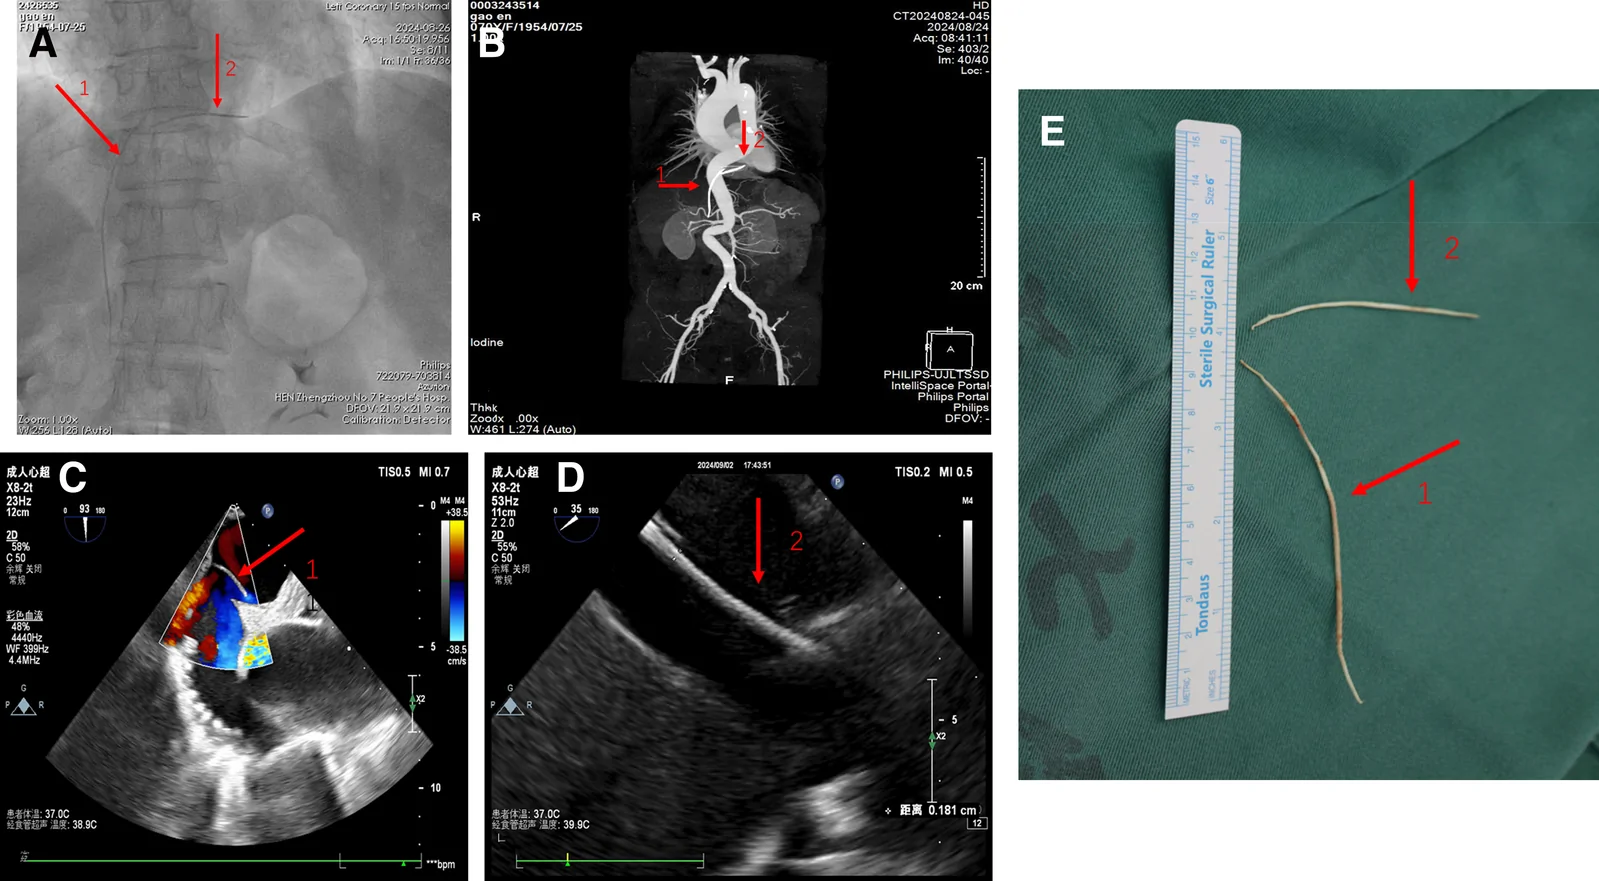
Multimodal imaging and intraoperative findings of intracardiac bone cement embolism. (A) IVC angiography showing bone cement fragments (arrows). (B) CT demonstrating linear high-density bone cement fragments within the IVC and right heart. (C, D) Transesophageal echocardiography showing a linear hyperechoic/striated foreign body extending through the right atrium and right ventricle, without definite pericardial effusion. (E) Intraoperative photograph of the two retrieved bone cement fragments. Arrow 1 indicates the bone cement fragment located at the entrance to the IVC, and arrow 2 indicates the fragment extending from the right atrium into the right ventricle and penetrating the right ventricular wall. Both fragments were removed under extracorporeal circulation, and the right ventricular wall was repaired. CT = computed tomography, IVC = inferior vena cava.
